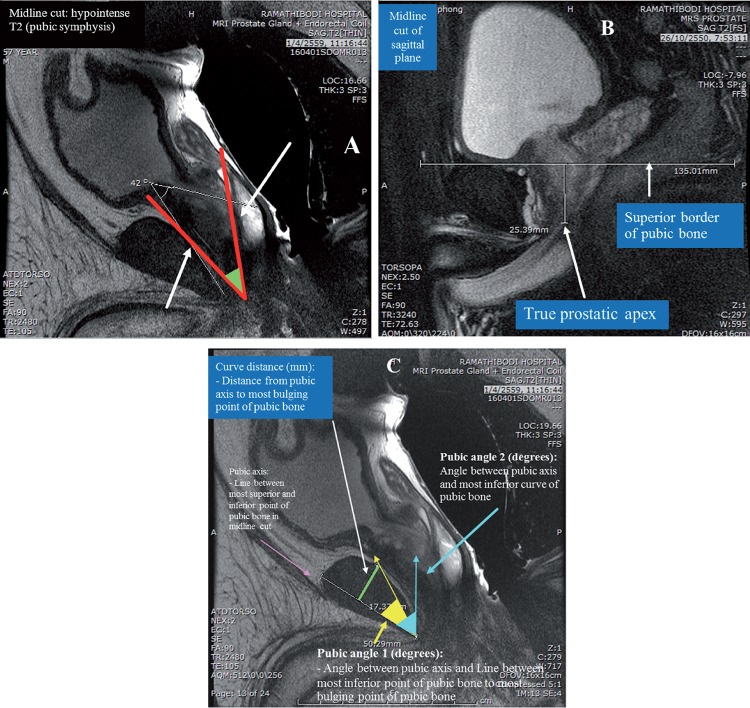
Anthropometric measurements by magnetic resonance imaging. (A) angle between the pubic bone and the prostate gland in midline cut: hypointense T2 (pubic symphysis); B) depth of prostatic apex; C) curve of public bone.
